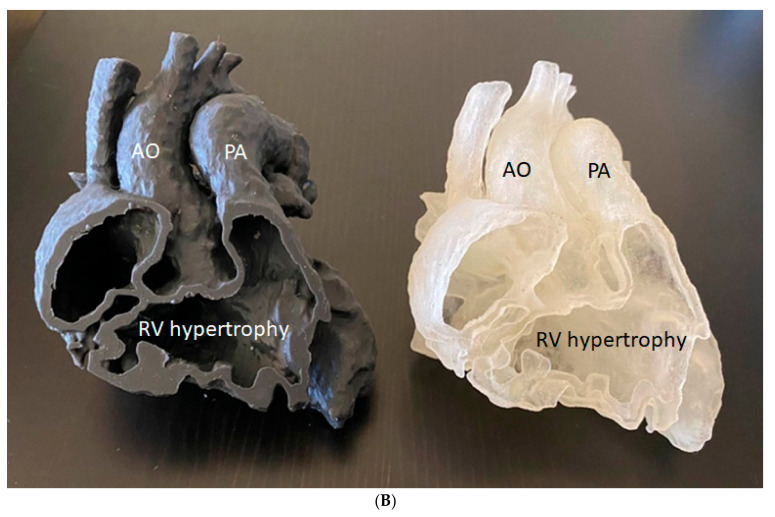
Three-dimensionally printed heart model of a patient with Tetralogy of Fallot. This model was printed based on cardiac CT images using Agilus30 material, and its tissue properties are similar to those of human heart tissues. The model was printed in one piece (A) and a two halves (B) to show the internal structures. The arrows refer to the pulmonary artery stenoses. AO—aorta, PA—pulmonary artery, RV—right ventricle. Reprinted with permission under open access from Sun [215].
